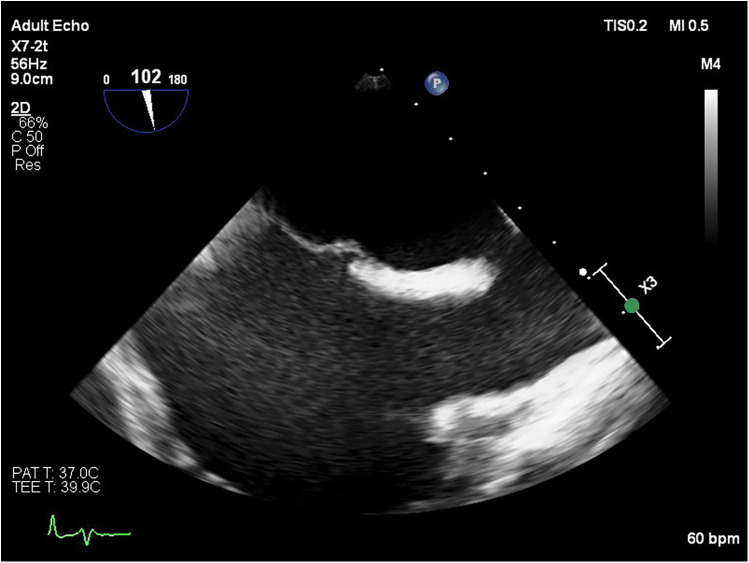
Transesophageal echocardiography image showing a sinus venosus atrial septal defect located near the junction of the SVC and the right atrium.
